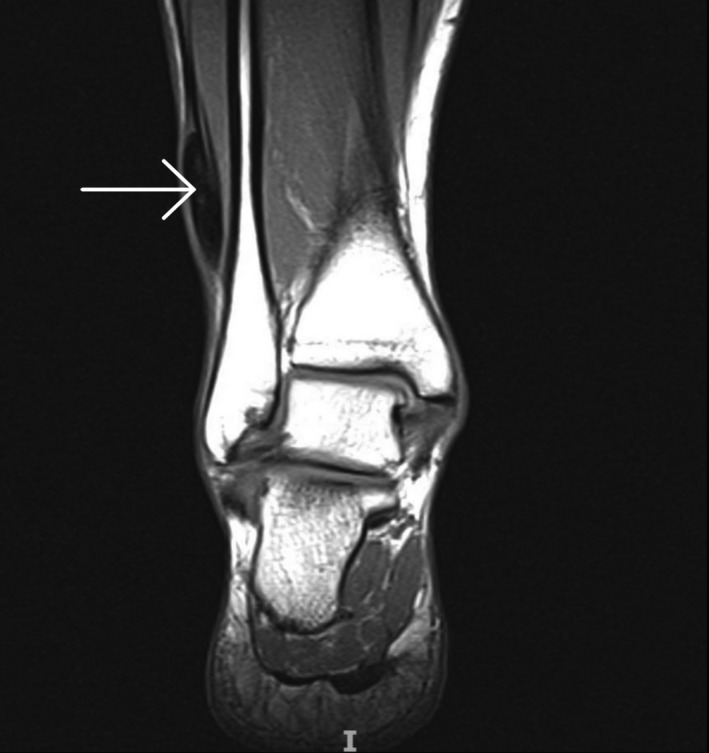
T1 sequence on the MRI showing a hypointense area of 3 × 1 cm in the medial lower extremity
